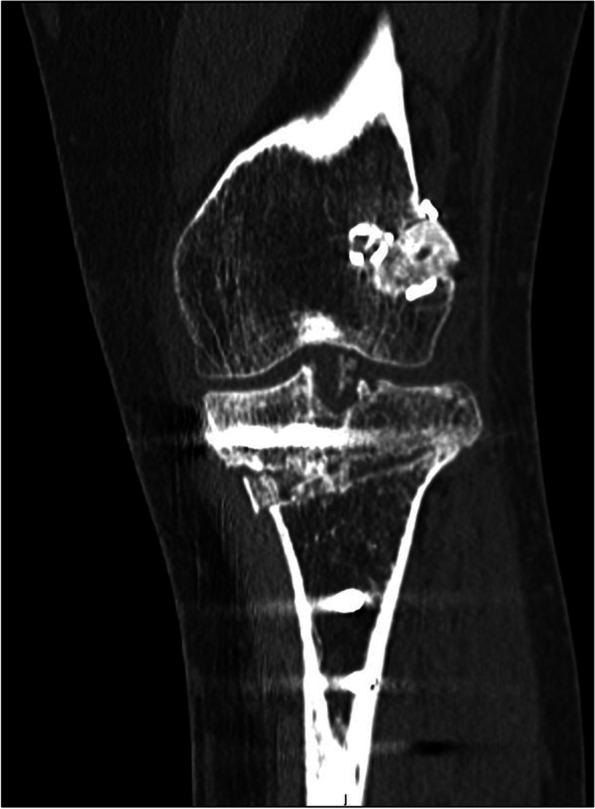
A coronal CT slice demonstrating delayed union of the osteotomy site 4 months post-operatively after the patient’s revision osteotomy and ACL reconstruction
